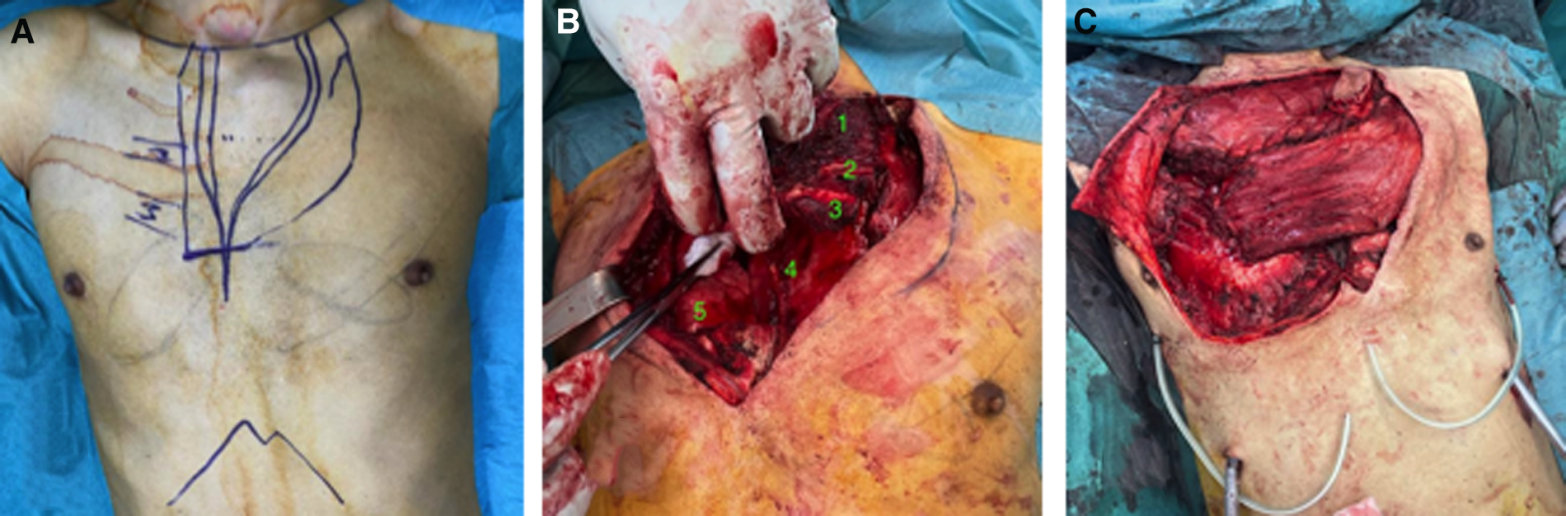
(A) Skin incision planning. (B) En-bloc resection of the manubrium sterni; upper part of the corpus sterni; first, second, and third bilateral parasternal ribs; and the medial clavicles, whose stumps were fixed on the first ribs. 1. Manubrium sterni tumor; 2. Second left rib; 3. Third left rib; 4. Mediastinal fat; 5. Right lung. (C) Musculocutaneous flaps covering chest wall reconstrauction.
